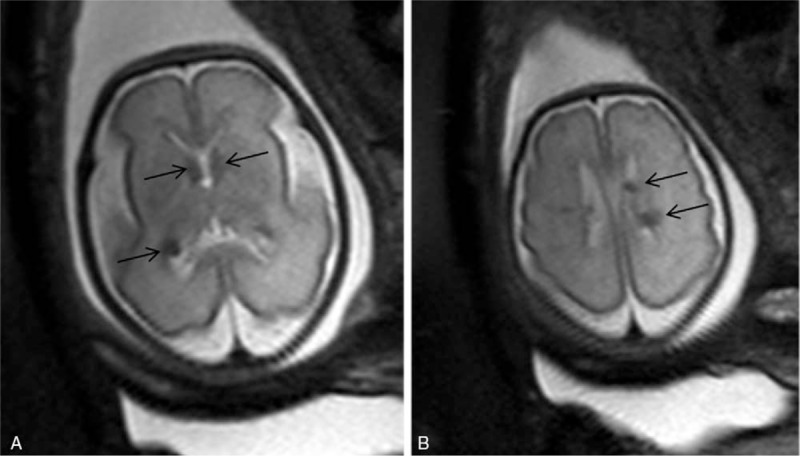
Cardiac rhabdomyomas were diagnosed by sonography in a 28 weeks gestation suggesting tuberous sclerosis but the fetal brain sonogram was normal. Axial T2 turbo-spin echo (TSE) single-shot magnetic resonance (MR) images show round hypointense (arrows) images located at the ependyma and adjacent to the Monro foramen, confirming Tuberous Sclerosis with cerebral lesions (A and B). These findings were confirmed at autopsy.
